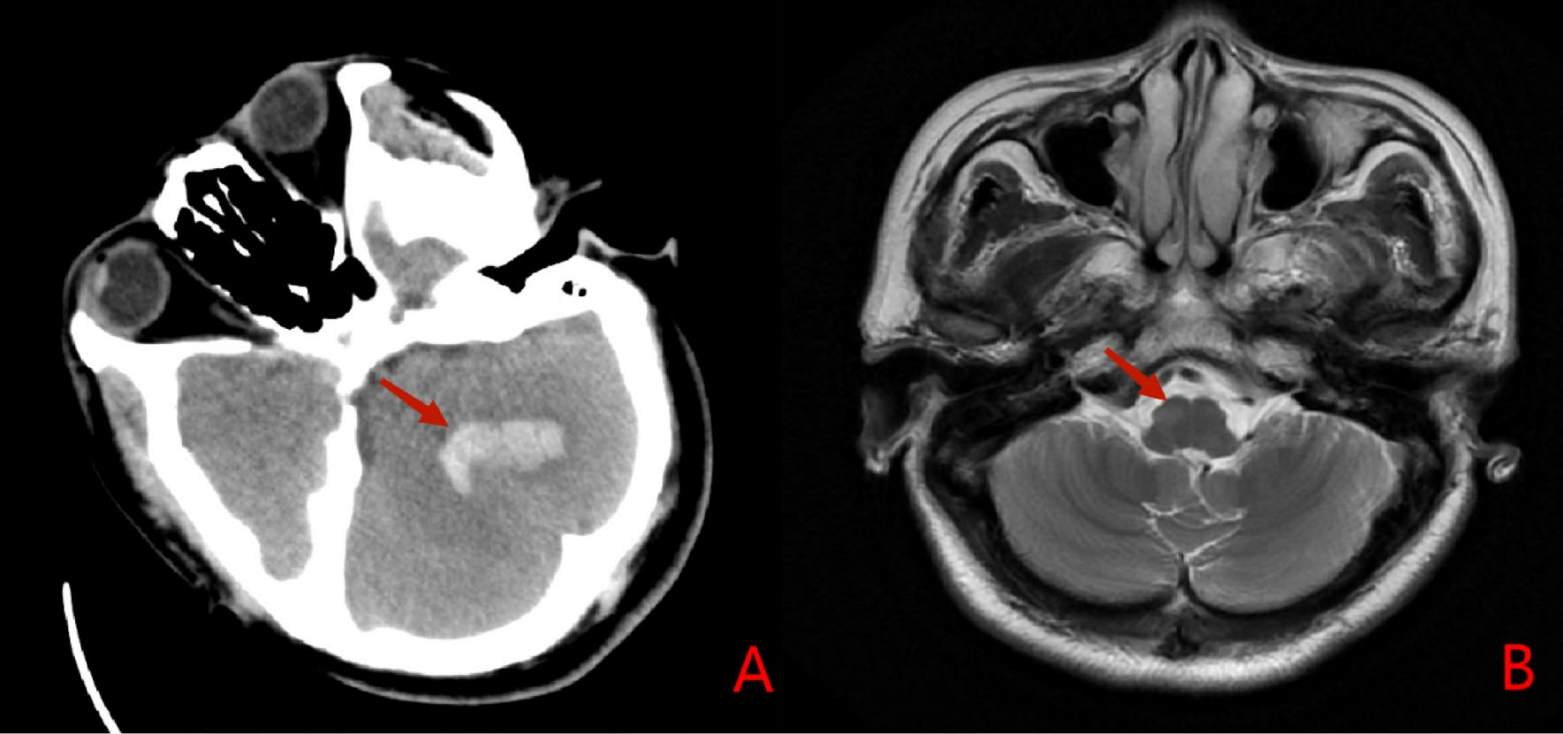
Case No.35, Head computed tomography (A) revealed cerebellar hemorrhage on the left side; T2WI (B) revealed hyperintensity on the contralateral ION 3 months after the insult.
